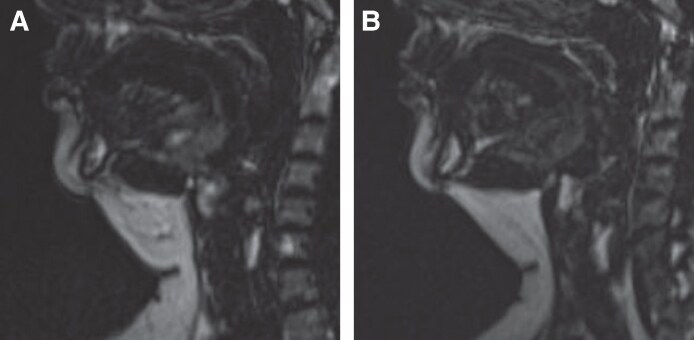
MRI scans of a 55-year-old female patient. This patient underwent combined HIFES and SyncRF+ treatment for submental volume reduction. (A) Baseline. (B) One month posttreatment with a reduction of submental fat layer (white color). The patient reported satisfaction with results, improvement in overall facial appearance, skin laxity, and jawline definition.
